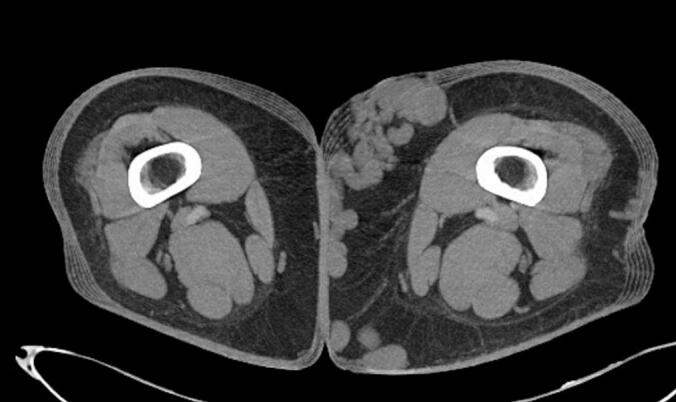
CT scan with widespread and significant varicose veins.
